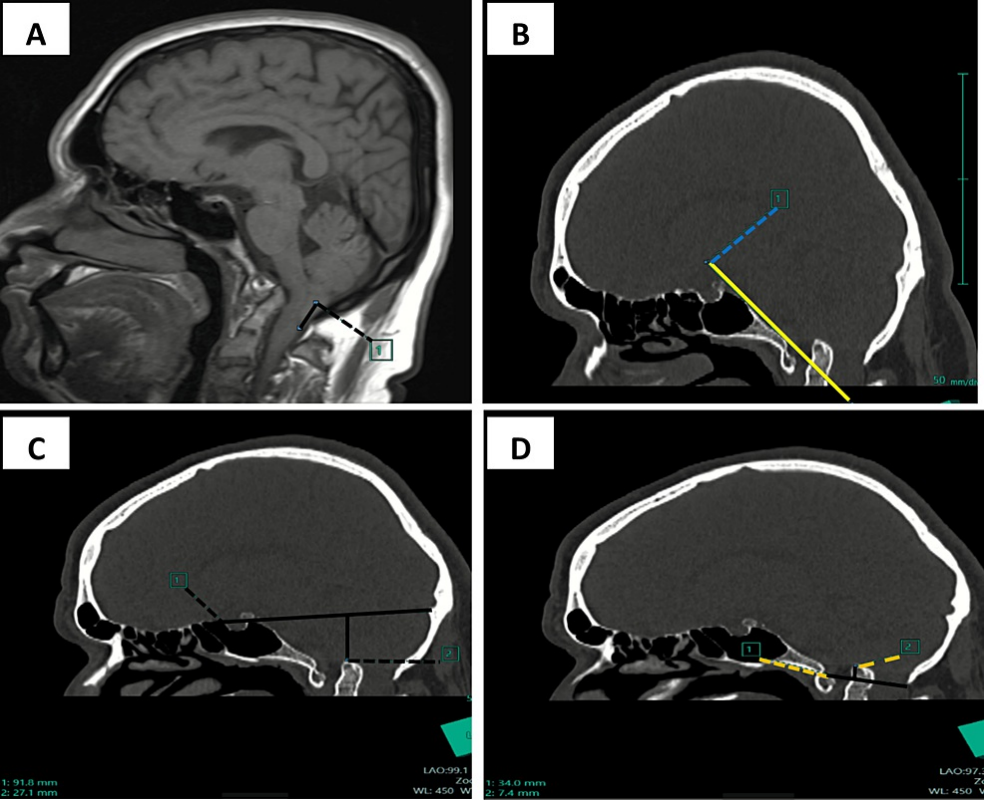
MRI showing caudal herniation of bilateral cerebellar tonsils noted for a distance of ~14.7 mm below the foramen magnum (A). Sagittal CT images show that Wackenheim’s line is intersecting the odontoid process (B). The height index of Klaus, which is the distance between the tip of the dens and the tuberculum torcula line, is 27.1 mm (C), McRae line, which is the tip of the odontoid process is ~7.5 mm above the line (D).
